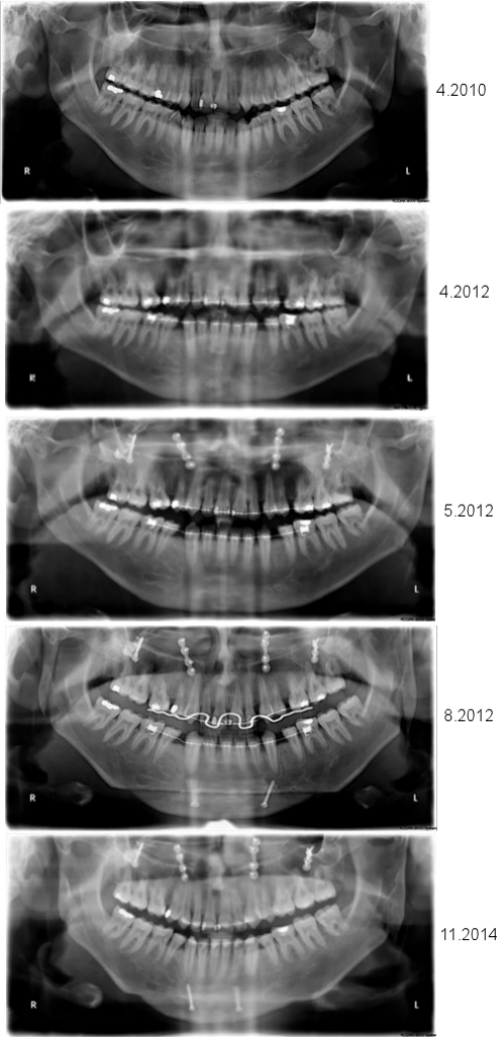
A series of orthopantomograms depicting the patient’s jaw during orthodontic-surgical treatment. From top to bottom: 1. situation prior to any therapy; 2. after orthodontics; 3. after maxillary osteotomy; 4. after mandibular osteotomies; and 5. after completion of post-surgical orthodontics.
